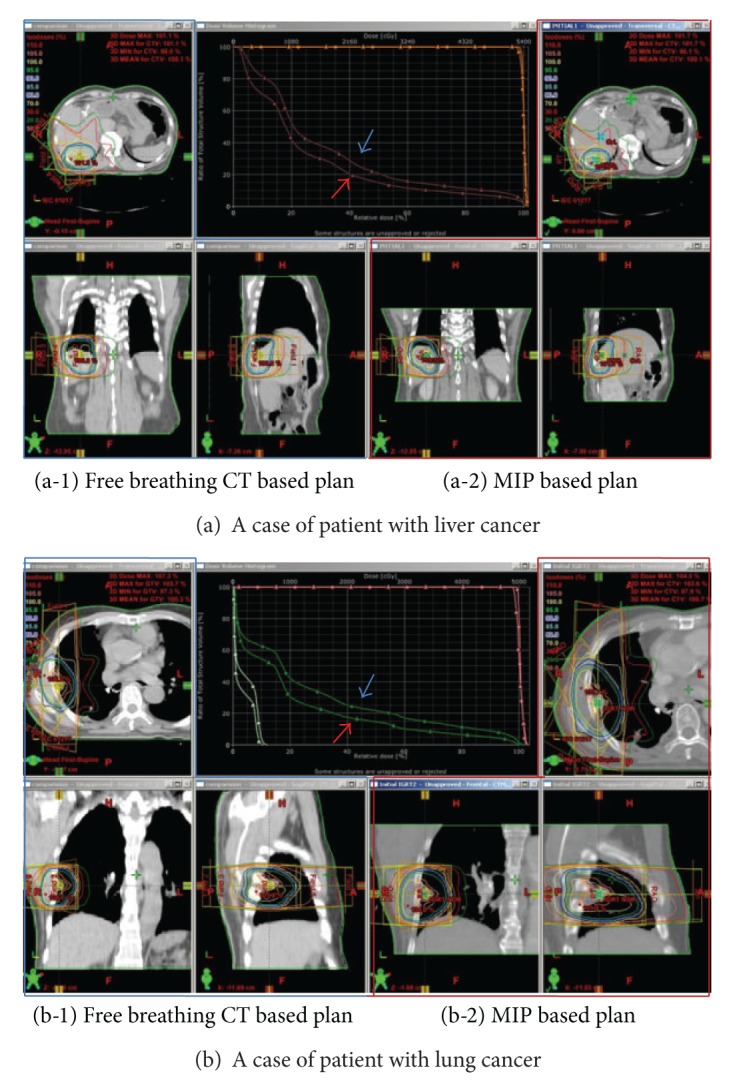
Comparison of dose volume histogram (DVH) of (a) liver and (b) lung between plans with free breathing CT (blue box and blue arrow) and 4D MIP CT (red box and red arrow) was shown.
